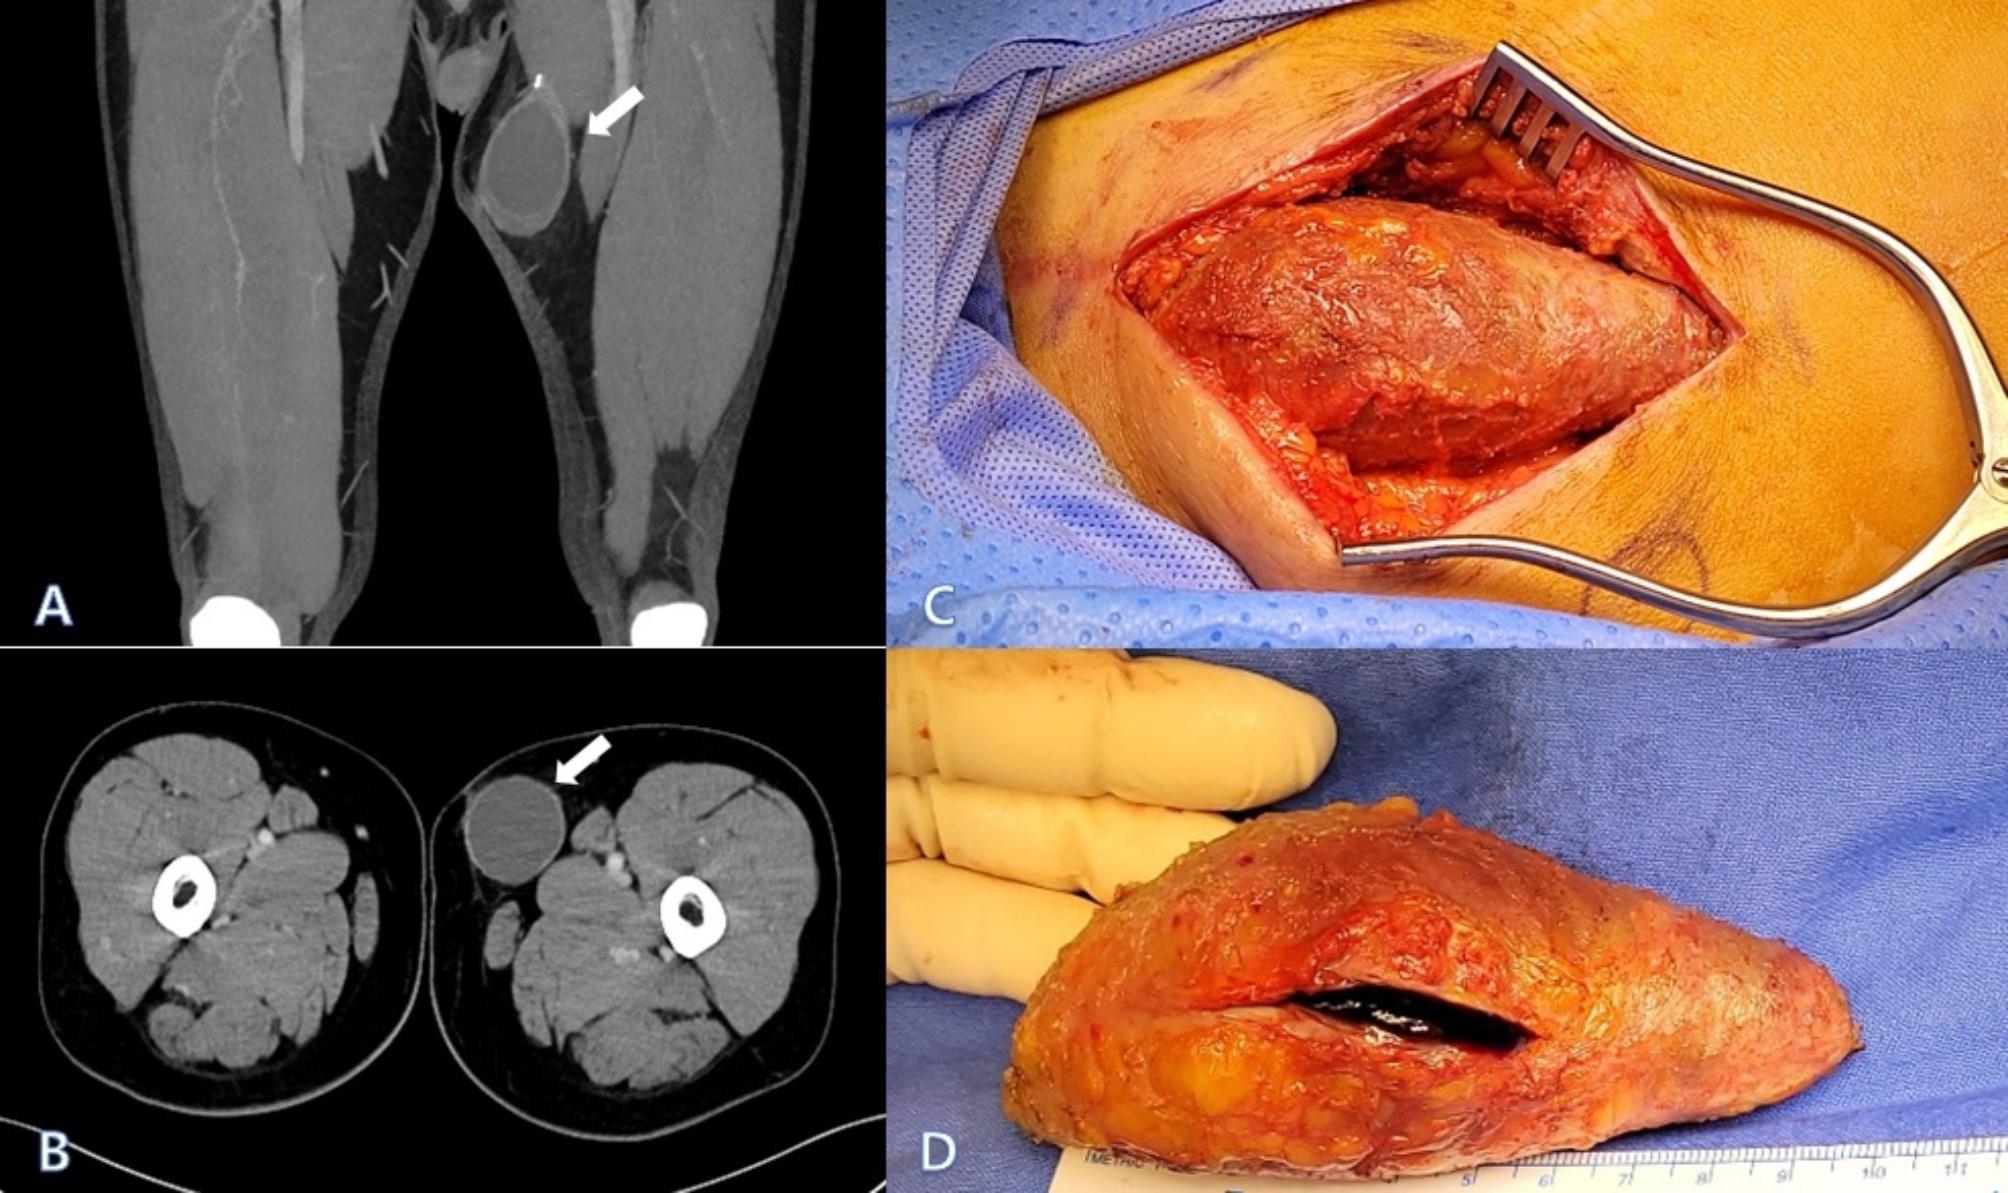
(A) Initial lower extremity CT revealing a capsulated mass implying hematoma or seroma (white arrow). (B) Axial view of the lower extremity CT showing the mass (white arrow). (C) Intraoperative findings showing a mass. (D) The thick fibrotic sac containing hematoma
